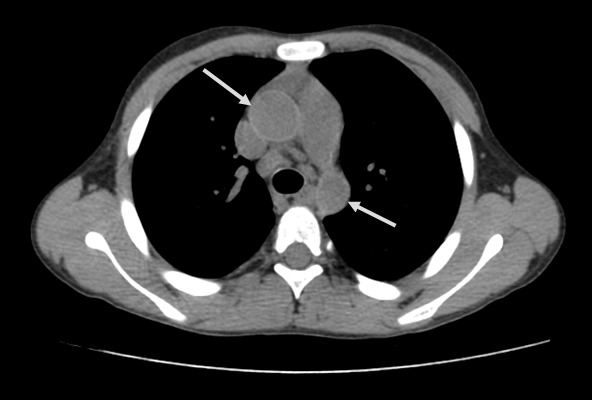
Chest computed tomography: anterior mediastinal mass on right and left main stem bronchi
